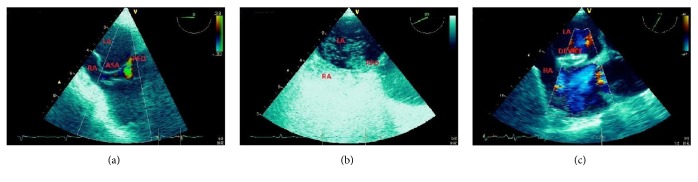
A 27-year-old male patient with patent foramen ovale (PFO) and a history of transient ischaemic attack (TIA). Patient had atrial device implantation. (a) Atrial septal aneurysm (ASA) with PFO and left-to-right shunt by transesophageal echocardiography. (b) TEE right-to-left shunt after intravenous injection of ultrasound contrast agent. (c) Postdevice transesophageal longitudinal bicaval view showing absence of shunt flow. LA = left atrium; RA = right atrium.
